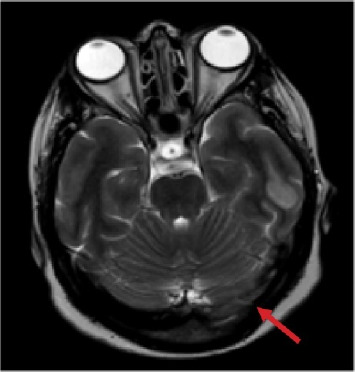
T2-weighted MRI scan demonstrating a signal abnormality in the left transverse (lateral) sinus, consistent with sinus thrombosis, which is marked by an arrow.
